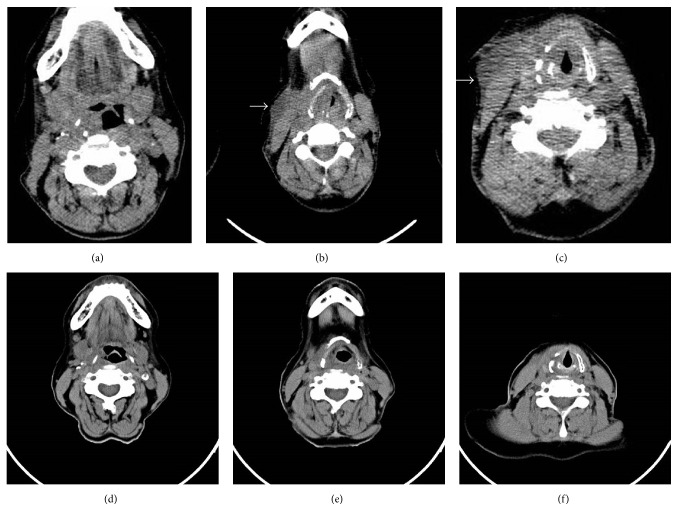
Cervical CT-scan of the laryngeal mass before (upper row: (a), (b), and (c)) and after (lower row: (d), (e), and (f)) radiotherapy.
